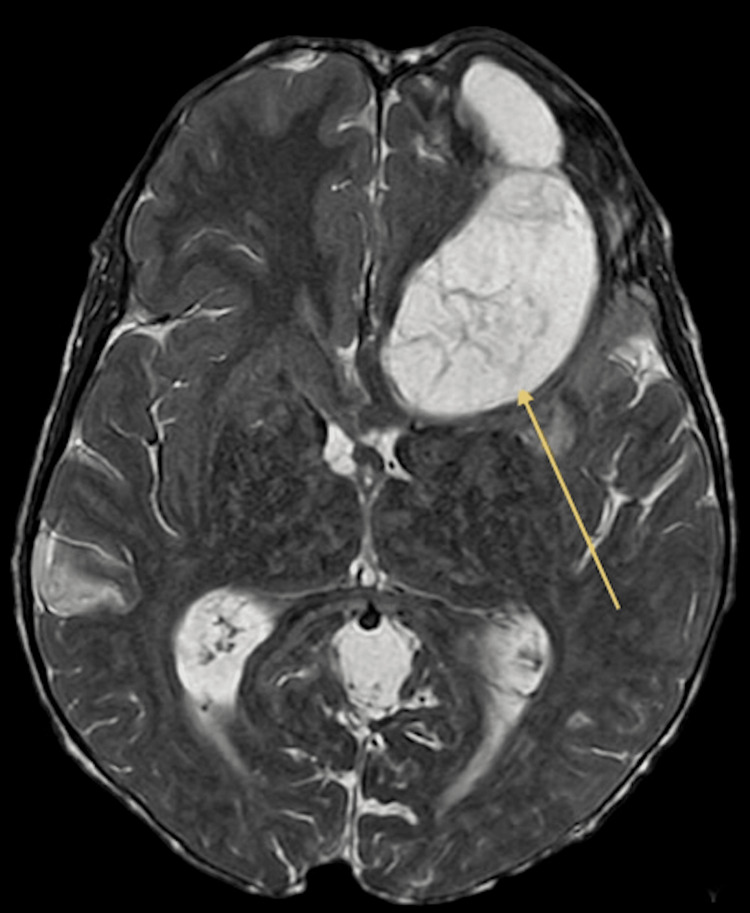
Contrast-enhanced MRI brain showing a hetero-intense bifrontal mass The yellow arrow points to the mass, projecting from the genu of the corpus callous with perilesional oedema and midline crossing.
